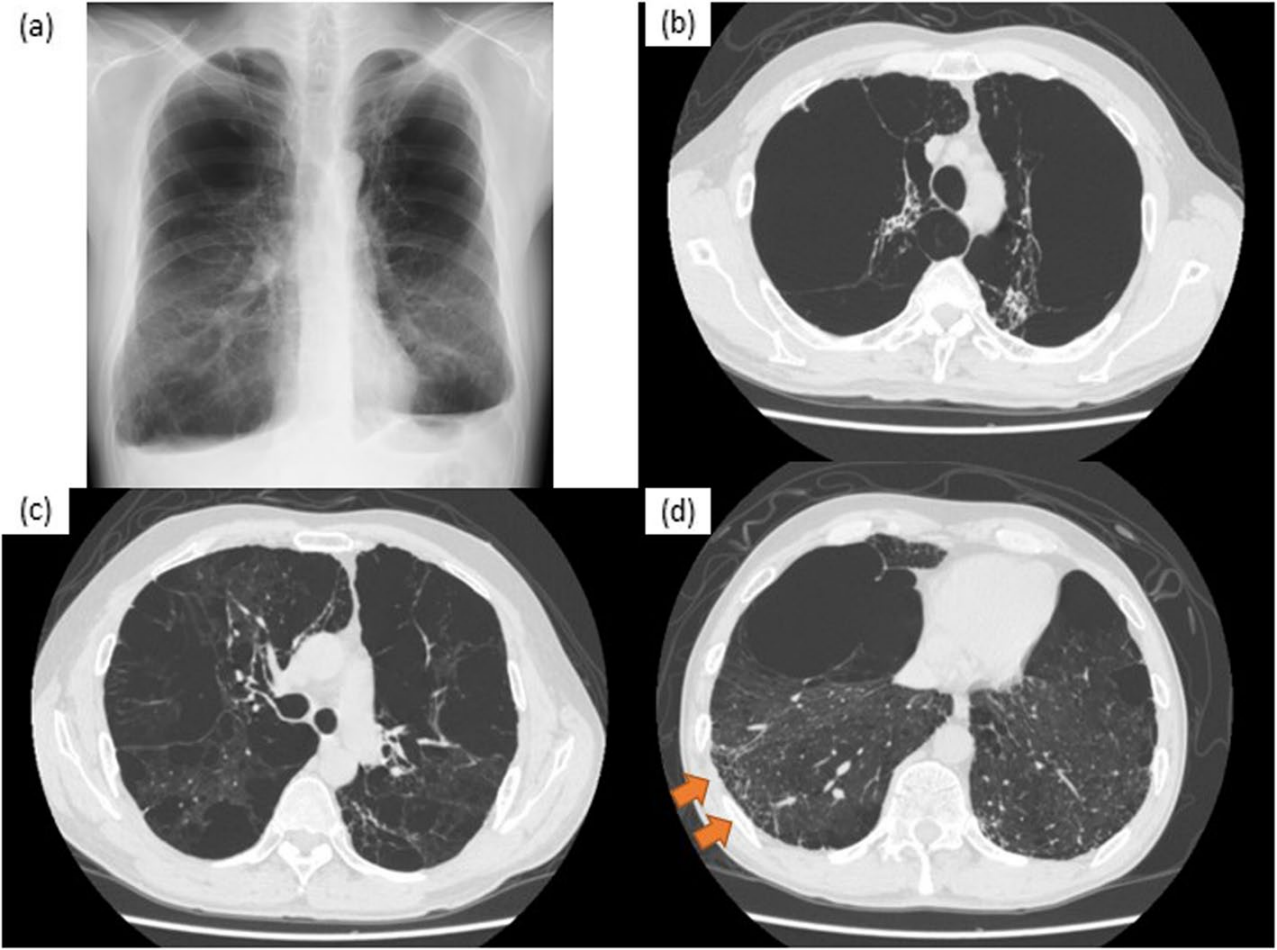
Images of chest X-ray and CT begore the lung transplantation. Images of chest X-ray (a) and CT (b)-(d). a The chest X-ray showed hyperinflation of bilateral lungs and a flattened diaphragm. b-d Cystic enlargement of airspace was more severe in the upper lobes than in the lower lobes. Reticular changes (orange arrows) were observed in the subpleural area in the area of ground-glass opacity mainly in the lower lobes
